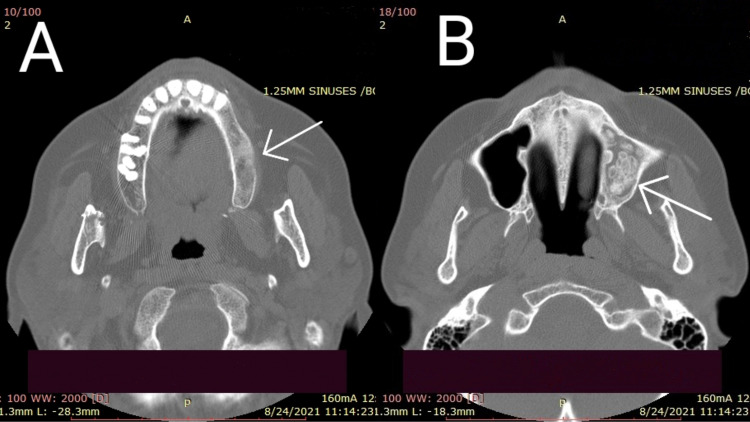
CT scan of the maxilla (A) Axial view showing the absence of the molar and premolar teeth on the left side compared to the right side, which showed normal growth of teeth. (B) Axial view showing hyperdense structures in the left maxillary sinus surrounded by soft tissue, representing the missing left premolar and molar teeth. CT, computed tomography
